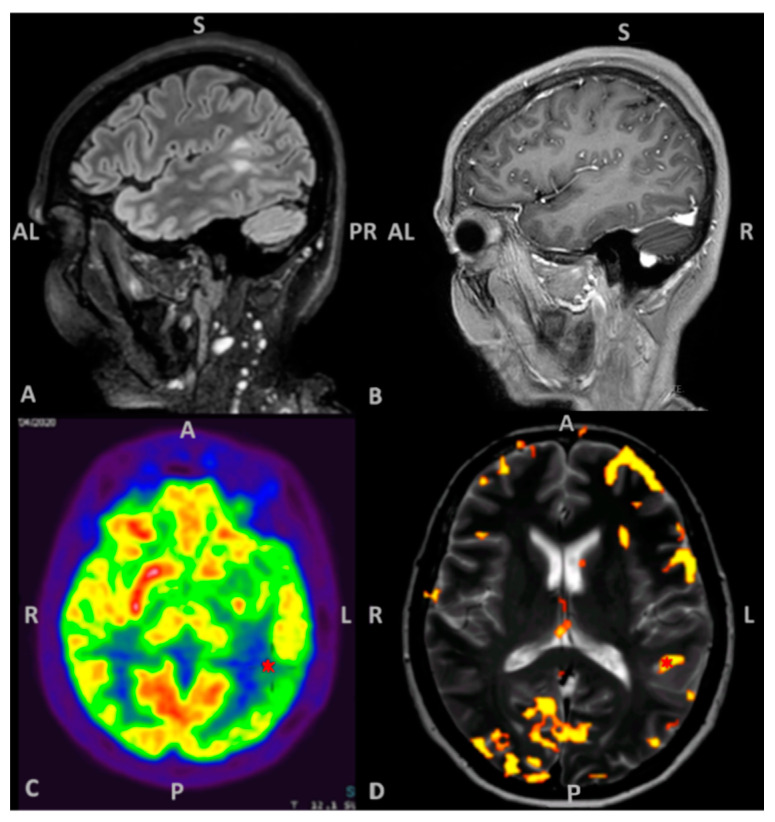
(A) 3D FLAIR (fluid attenuated inversion recovery) image discloses a primitive brain tumor located between the posterior part of the superior and middle temporal gyri and the anterior part of the angular gyrus in the left hemisphere; (B) the lesion does not enhance after contrast medium administration; (C) the 18F-fluorodeoxyglucose PET/MRI reveals the hypometabolism of the tumor (*). (D) Axial T2w image fused with the BOLD (blood oxygenation level dependent) signal activation map obtained during word generation task shows a focal cortical activation in the superior–anterior part of the lesion (*).
